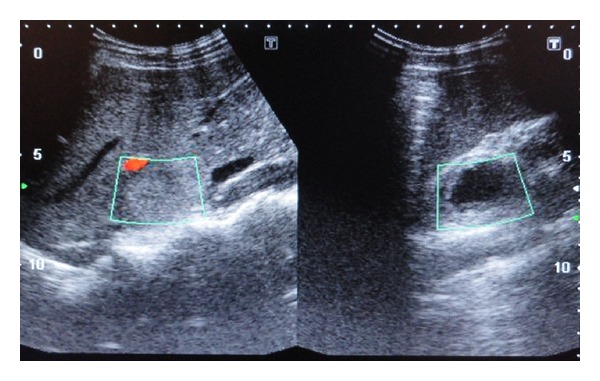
Ultrasound of abdomen showing heterogeneous lesions at the upper poles of both kidneys suggestive of bilateral adrenal masses.
